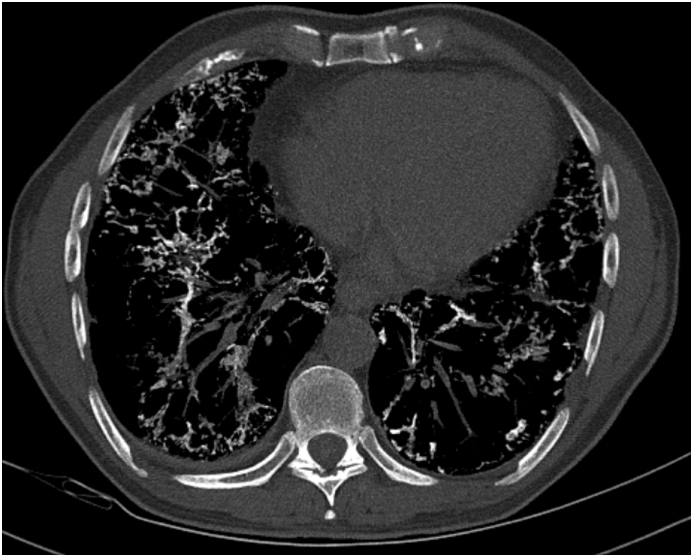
Transverse cut of a high resolution computed tomographic scan of the chest on inspiration using maximal intensity projection using bone windowing demonstrating the diffuse parenchymal calcifications throughout the bilateral lower lobes of the lungs.
